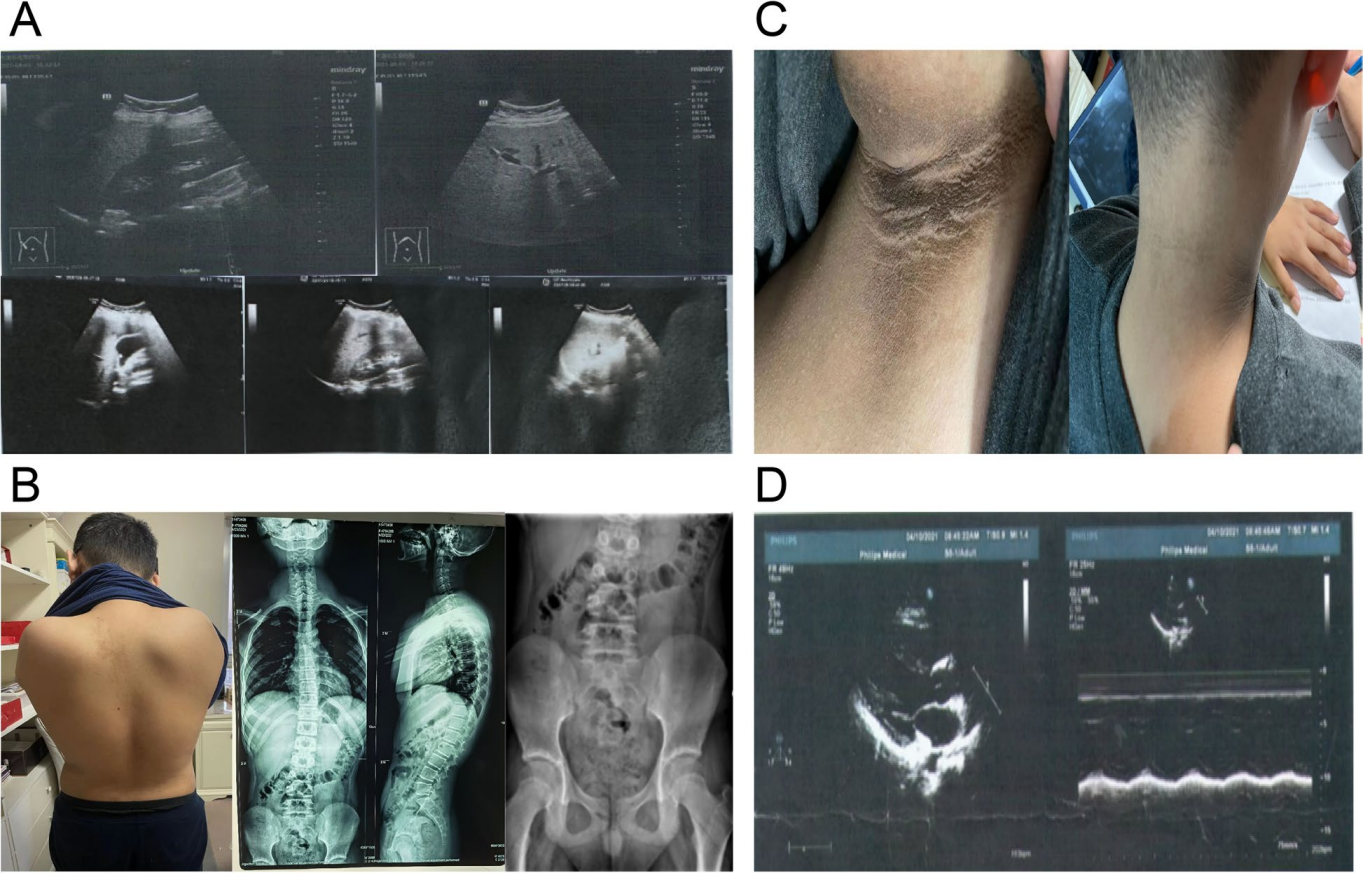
Ultrasonic examination and echocardiography. A Results of ultrasound examinations. The two images at the top are from 2021, and the three images at the bottom are from 2022. B Development of scoliosis and epiphysis. C Presence of acanthosis nigricans. D Echocardiography findings
